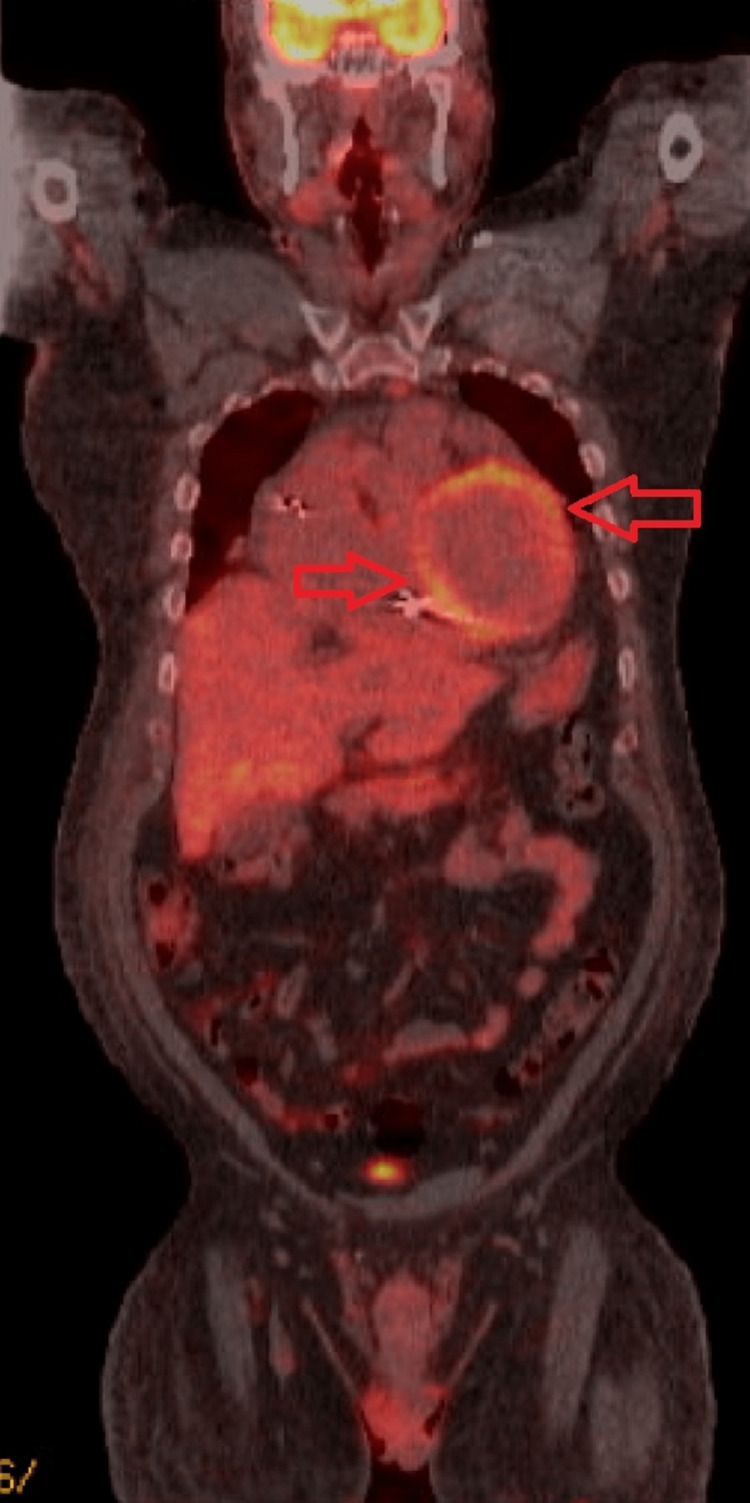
PET scan coronal view revealing diffuse FDG uptake in the heart PET: positron emission tomography; FDG: fluorodeoxyglucose
